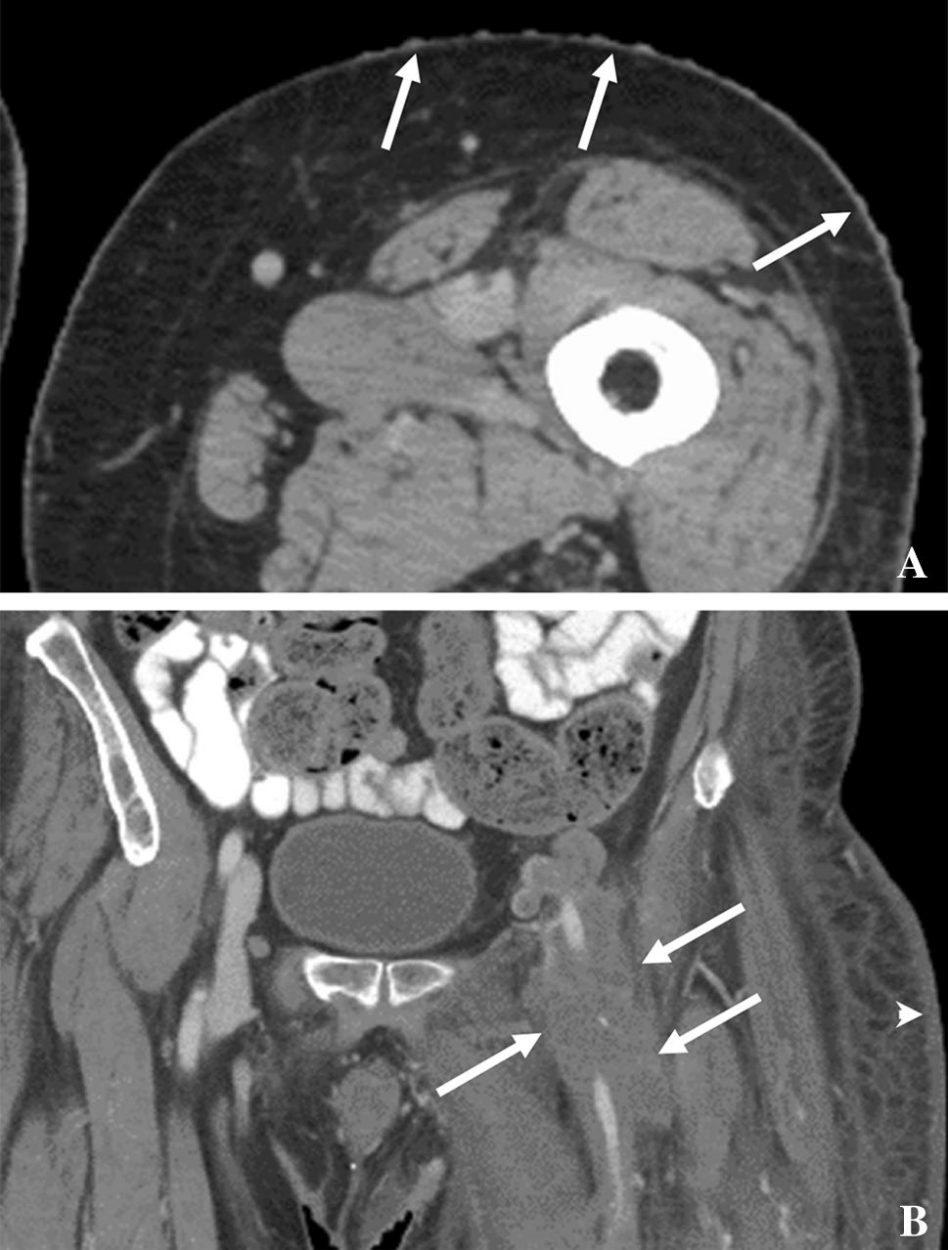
A A 56-year-old man with HIV and KS. Contrast-enhanced axial CT of left thigh showing nodular areas of skin thickening consistent with KS (arrows). B A 58-year-old man with HIV and KS. Contrast-enhanced coronal CT showing multiple enlarged nodes encasing the left common femoral artery (arrows) consistent with KS. Skin thickening in left gluteal region (arrowhead) is due to skin involvement by KS. Note: These images are of the same patient but from different years
